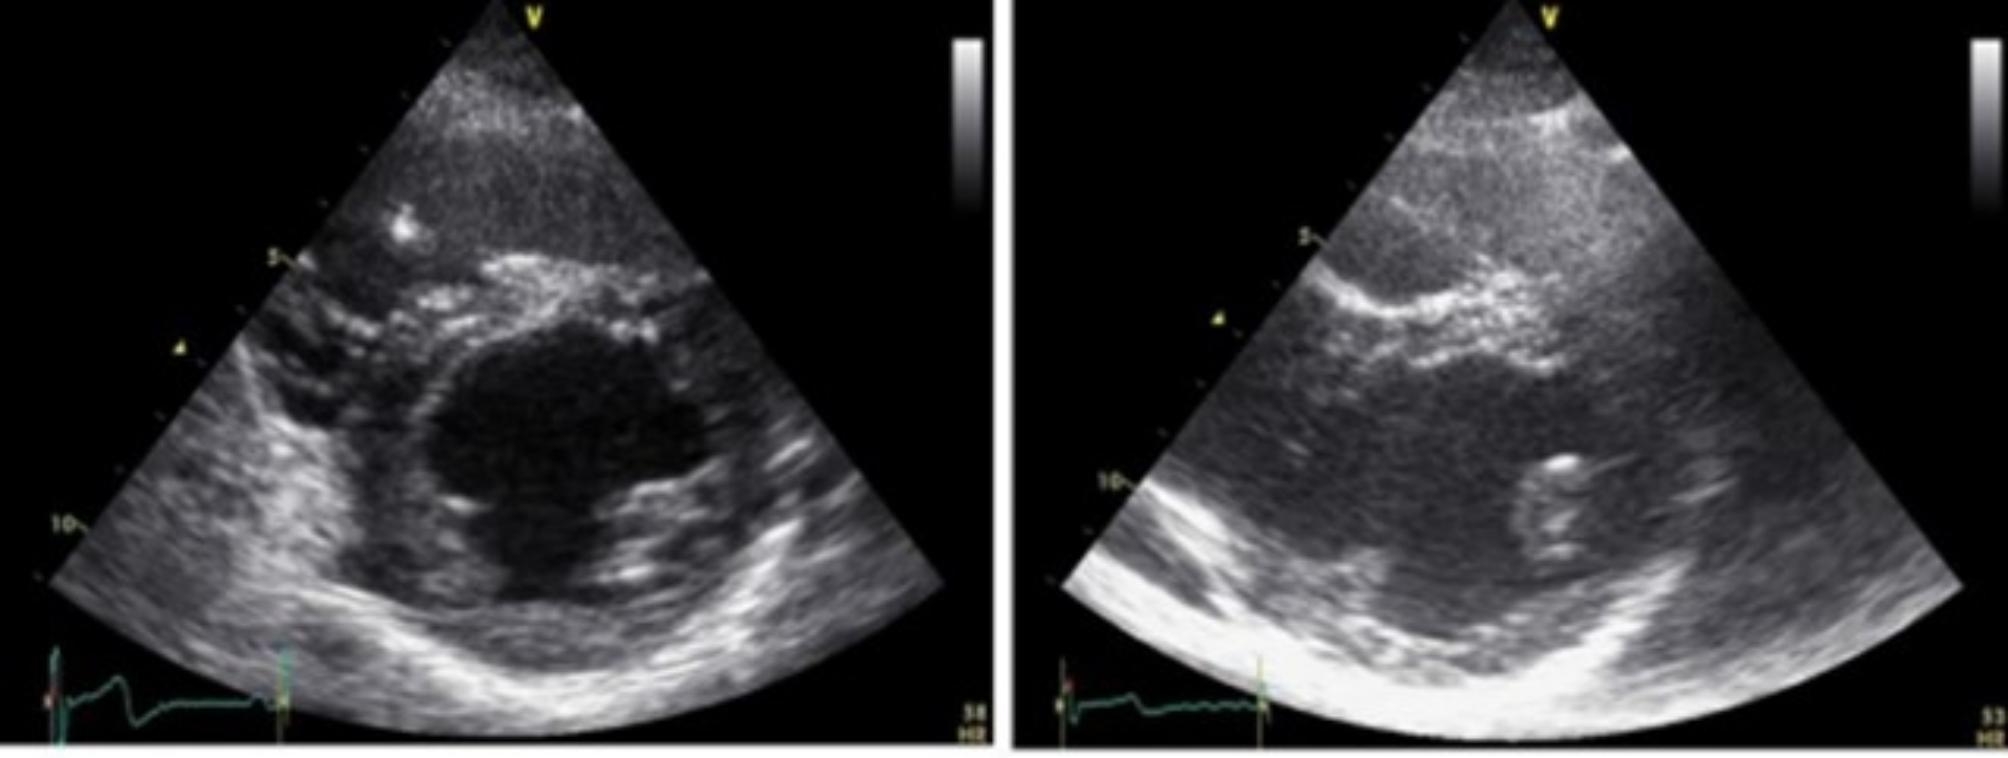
Patient with Fabry disease without left ventricular hypertrophy. Two-dimensional Echocardiogram. Left parasternal short axis at end-diastole. Left panel: shows normal posteromedial and anterolateral papillary muscles. Right panel: shows hypertrophy of the anterolateral papillary muscle, without left ventricular hypertrophy
